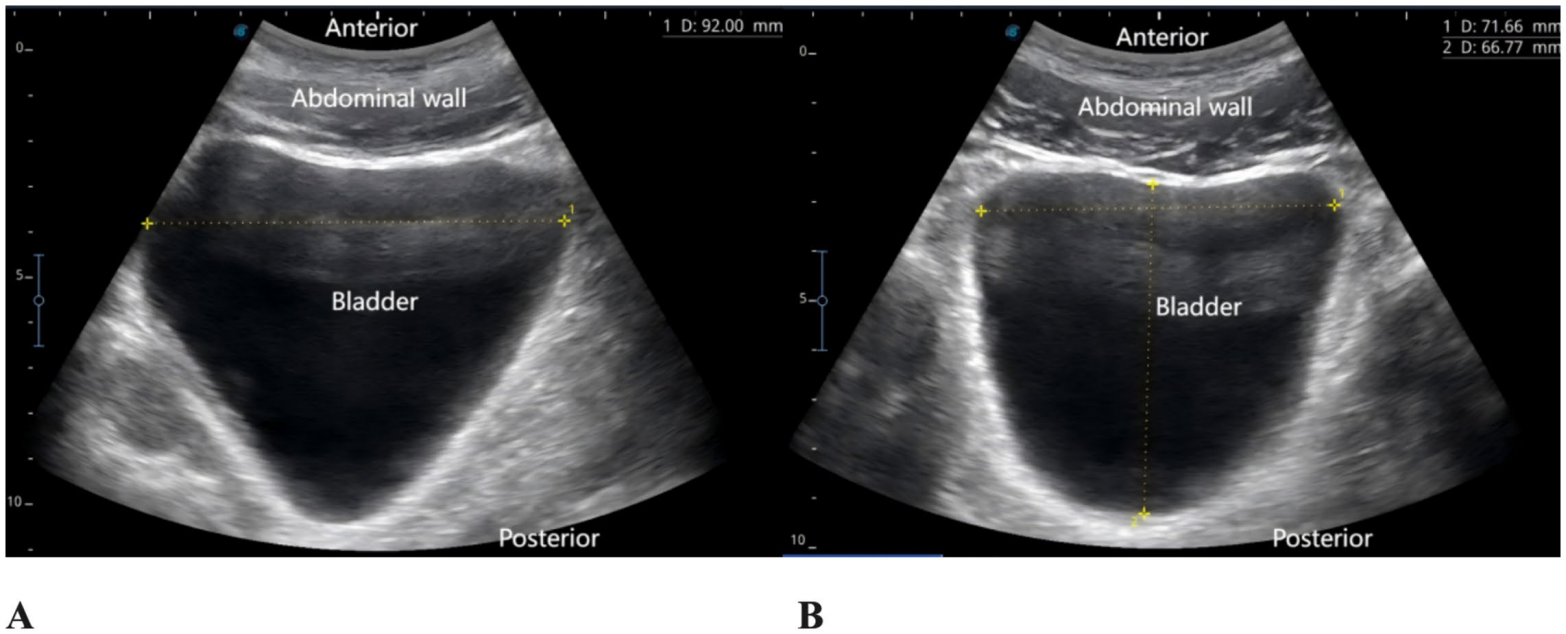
Bladder ultrasound images. (A) Sagittal view. (B) Transverse view.
